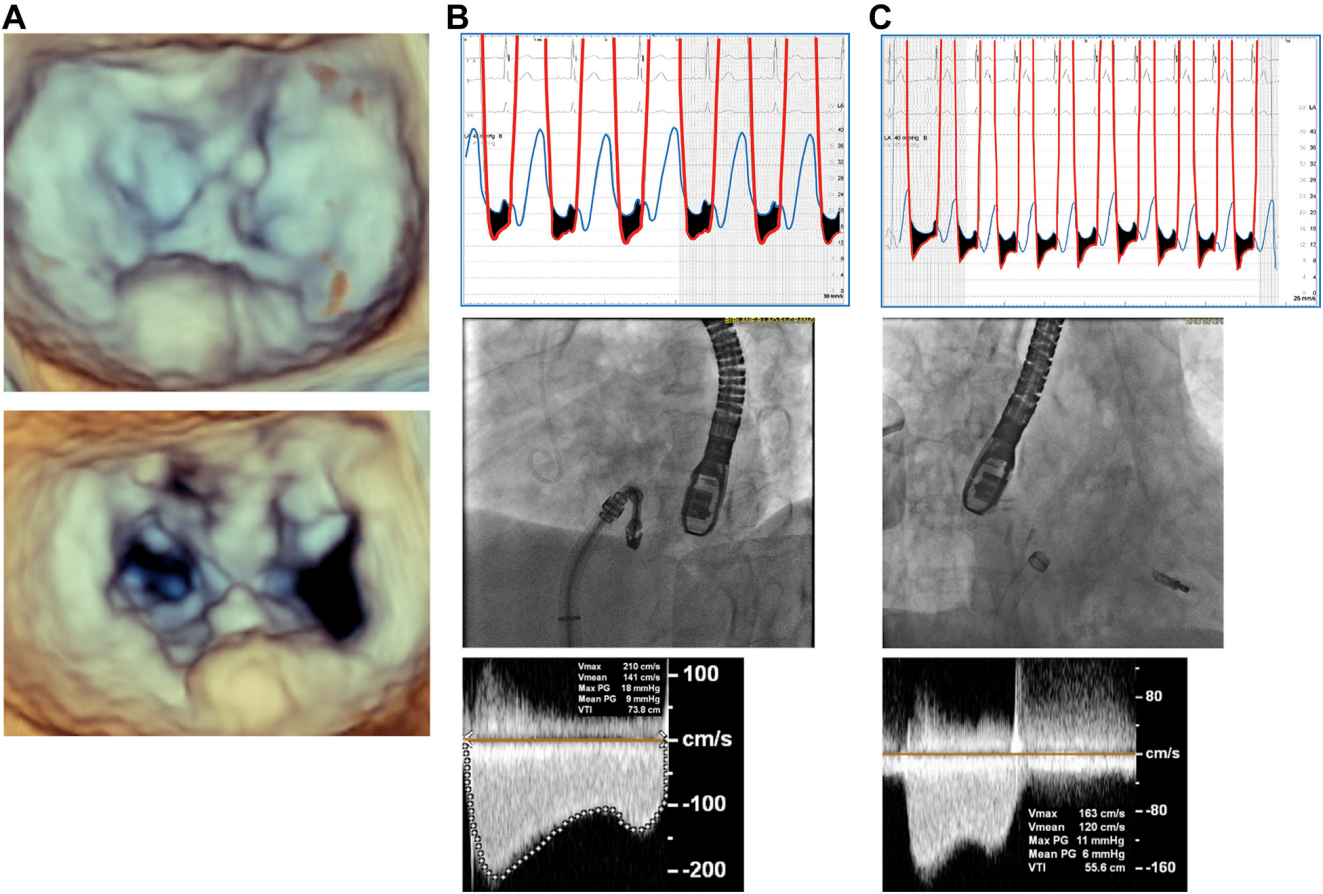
Three-dimensional echocardiographic mitral valve reconstruction and hemodynamic data. (A) Pre-MitraClip and post-MitraClip 3-dimensional echocardiographic reconstruction of the mitral valve, demonstrating severe posterior mitral annular calcification. (B) Hemodynamics after grasping both leaflets with the NT MitraClip device still attached to the guide. (C) Hemodynamics after retraction of the guide into the right atrium after deployment.
